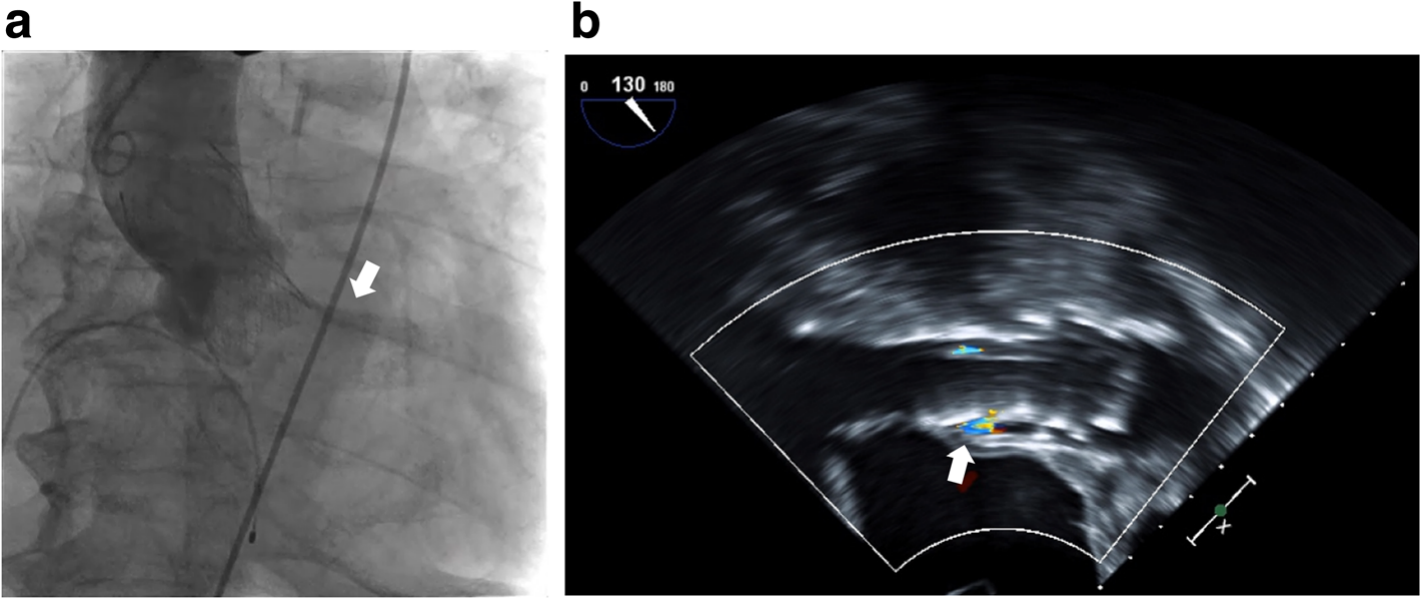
Immediate post-procedural findings. a The aortogram revealed mild paravalvular aortic regurgitation (arrow). b Transesophageal echocardiography showed mild paravalvular aortic regurgitation (arrow)
